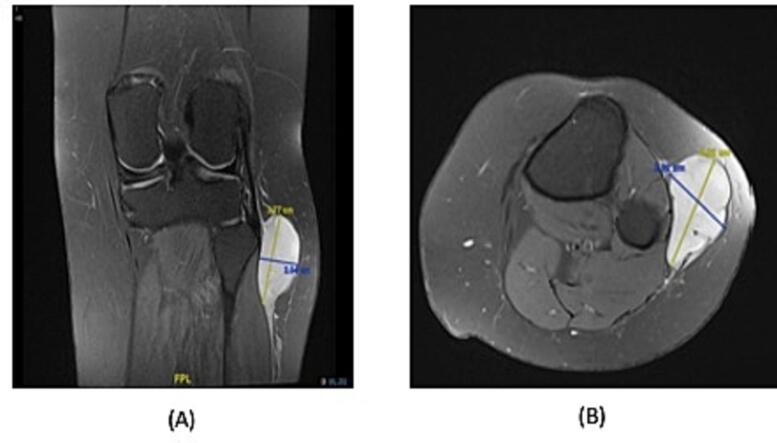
This is a contrast-enhanced MRI of the lesion. (A) The coronal view revealed a cystic lesion approximately 3.7 × 2 cm in the lateral head of gastrocnemius, adjacent to the common peroneal nerve. (B) The axial view revealed a cystic lesion approximately 4 × 2.9 cm in the lateral head of gastrocnemius.
